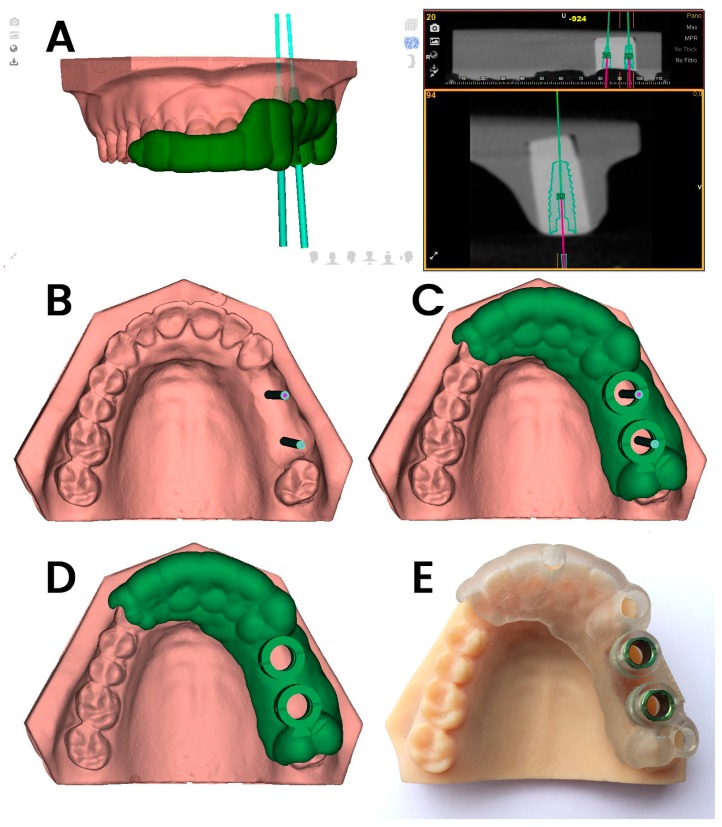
(A–C) Dental implant planning with the computer-aided static navigation system using a cone-beam computed tomography (CBCT) scan (green lines); (C,D) virtual template design according to the planned virtual dental implant placement; (E) manufactured stereolithography template fixed over the dental surface of the teeth and placed over the partially edentulous upper jaw models.
